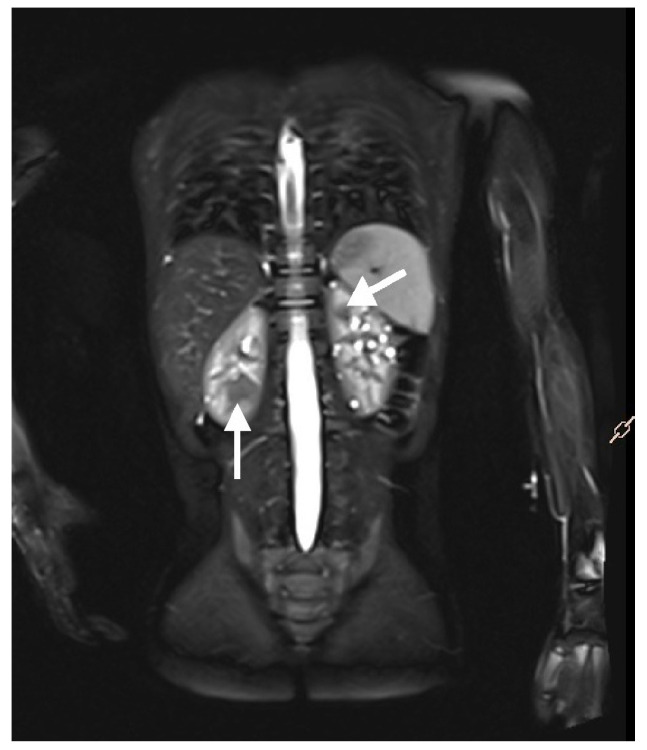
An MRI with contrast of a 5-year-old boy. Multiple solid focal lesions in both kidneys—atypical angiomyolipomas (arrows). Additionally, numerous small cysts within the parenchyma of both kidneys.
